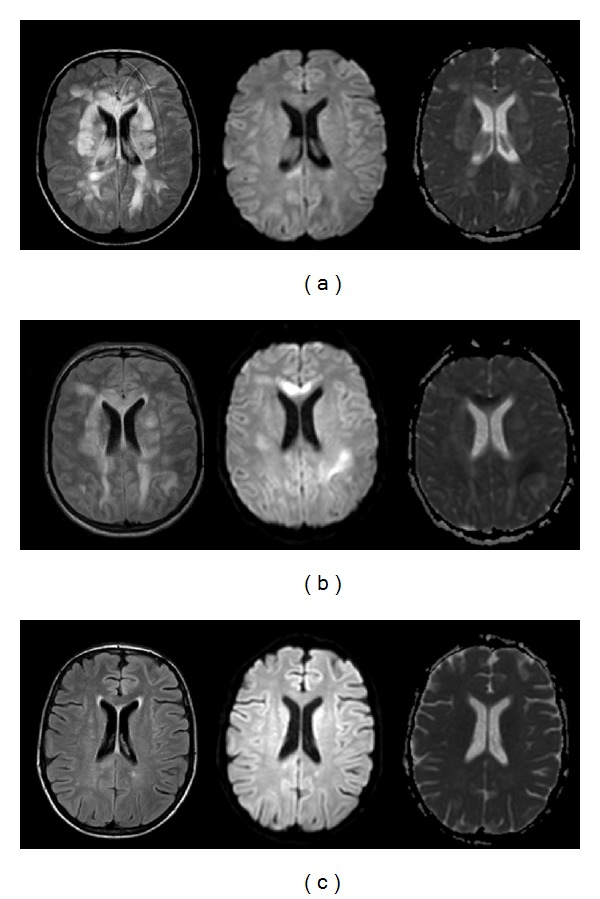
MRI evolutions in Patient 1. (a) Deep white matter vasogenic edema. (b) Incomplete regression of vasogenic edema with cytotoxic edema in corpus callosum and parietal regions. (c) A remarkable resolution of vasogenic/cytotoxic edema.
